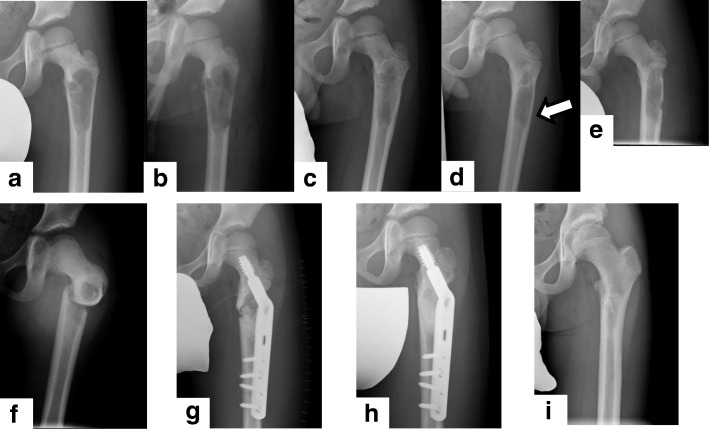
Recurrence and pathologic fracture after second endoscopic curettage. A 5-year-old boy had left coxalgia; from the X-ray image (a), simple bone cyst was suspected. The first endoscopic curettage was performed via two portals (b). After 2 months, bone healing had begun with cortical enlargement and consolidation of cancellous bone (c). However, approximately 1 year after the endoscopic curettage, the cystic lesion (white arrow) had become prominent and recurrence was suspected (d). A second endoscopic curettage was performed (e). After discharge with a crutch, the patient fell, and a subtrochanteric fracture was identified (f). Open reduction and internal fixation with a compression hip screw (Ti-VFx II tube plate®, Zimmer Biomet, Warsaw, USA) and artificial bone grafting (OSferion®, OLYMPUS) were performed (g), and after 6 months, bone union was confirmed (h) and the implants removed. Three years after the first endoscopic curettage, no recurrence or complications had occurred (i)
